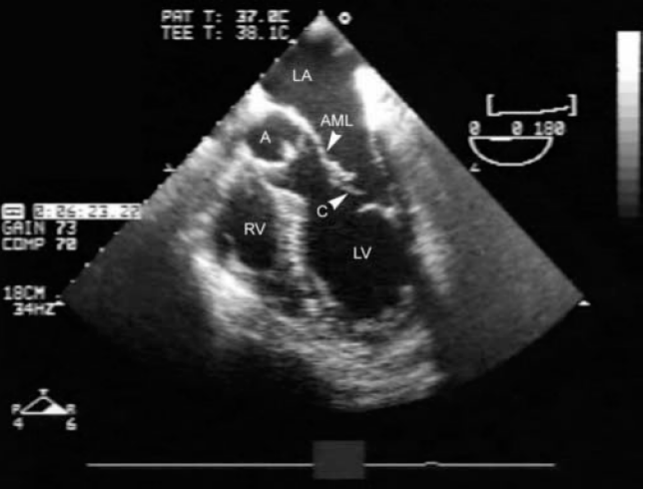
Transesophageal Echocardiogram showing ruptured chordae (C) of anterior mitral leaflet (AML). Left ventricle (LV) and Left atrium (LA), Right ventricle (RV) and aortic valve (A) are shown.
